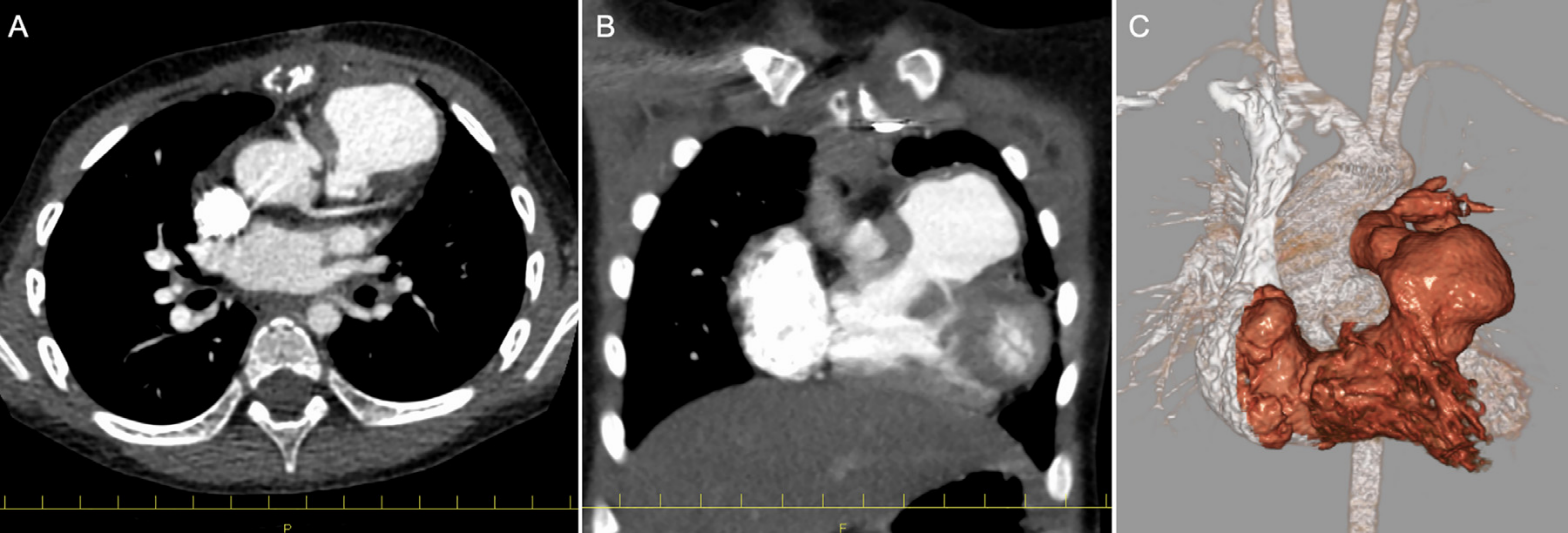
(A-C) Contrast-enhanced computed tomography revealed aneurysm formation in the RVOT.
